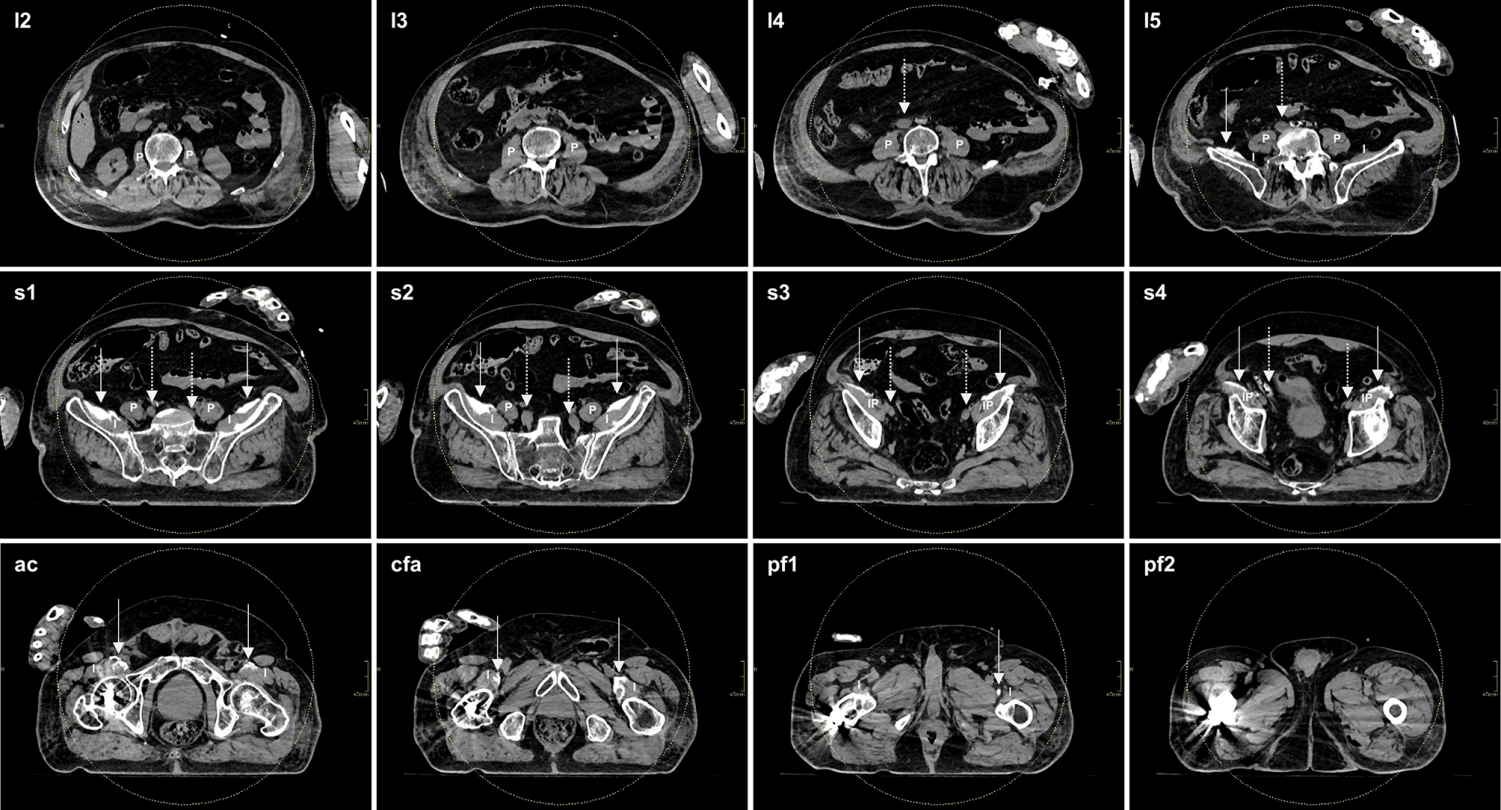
Axial CT slices detailing typical spread of injectate after (bilateral) S-FICB-H. The LA-based contrast mixture was determined to reach a maximum height corresponding to the fifth lumbar vertebra on the left side and the intervertebral disc of lumbar vertebrae four-five on the right side. Medial spread of contrast stayed anterior to the iliopsoas muscles and iliac arteries. As such, contrast remained removed of the location of the lumbar plexus and ON, both in the cranial and medial directions. Dotted arrows point to the iliac arteries, solid arrows point to the contrast. *l* lumbar vertebra, *s* sacral vertebra, *ac* acetabulum, *cfa* common femoral artery, *pf* proximal femur, *P* psoas muscle, *I* iliacus muscle, *IP* iliopsoas muscle.
